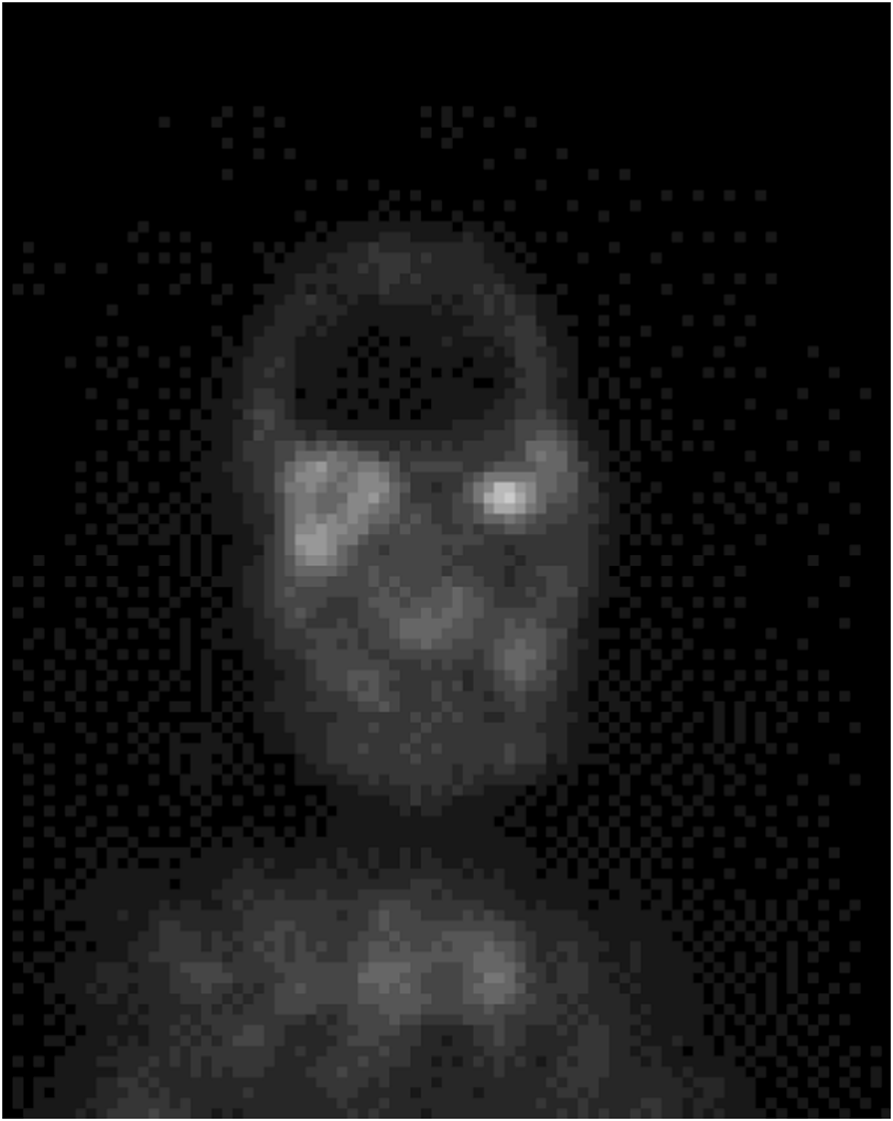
Radioisotope inflammatory scintigraphy image. Intense uptake of gallium-67 in the bilateral but right side-dominant orbital lesion.
